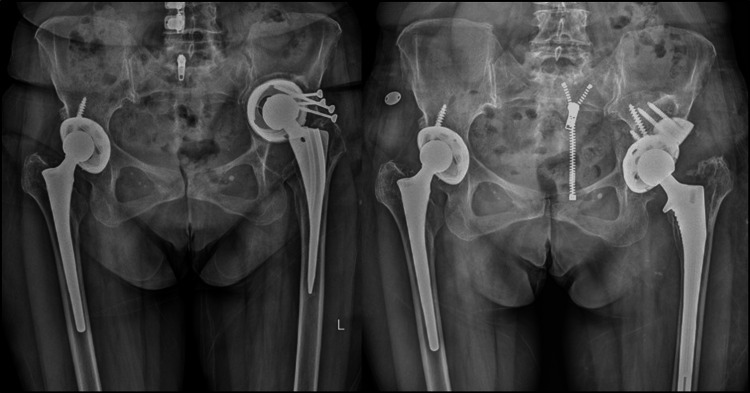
Revision THA with the use of metal augment in 62-year-old female patient indicated for proximal and medial migration of acetabular component (Paprosky defect type IIIa) X-ray before surgery (left); X-ray one year after surgery (right) showing adequate osseointegration of acetabular component and augment THA: total hip arthroplasty Image credits: Zelimir Jovanovic (author)
